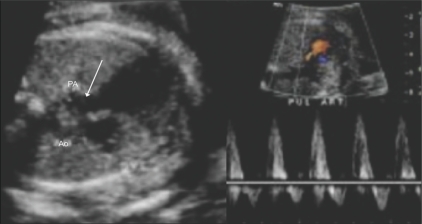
Pulmonary stenosis. A three-vessel view (A) shows pulmonary artery stenosis (arrow); there is a prominent aorta (Ao) since the patient also had a VSD. The color Doppler image (B) shows high peak velocities with aliasing. PA – pulmonary artery.
